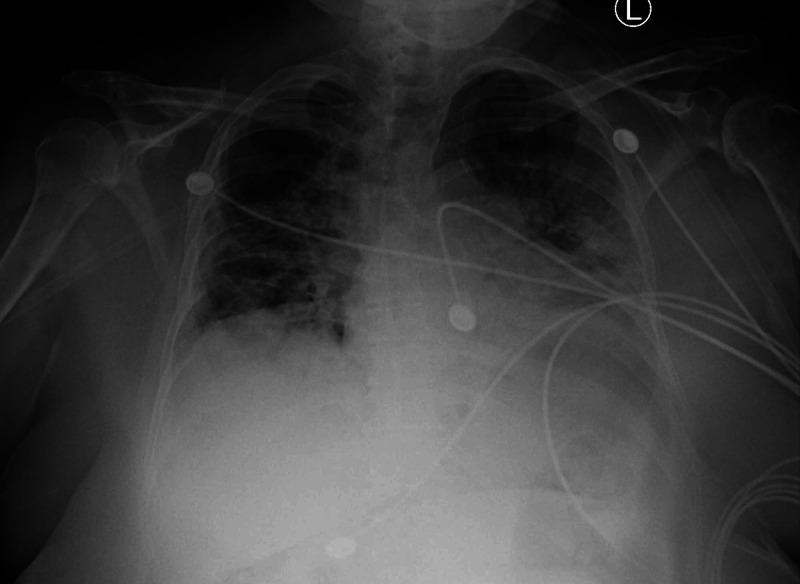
Anteroposterior (AP) X-ray of the chest upon admission X-ray demonstrating bilateral hazy opacities suspicious for a viral pneumonia
